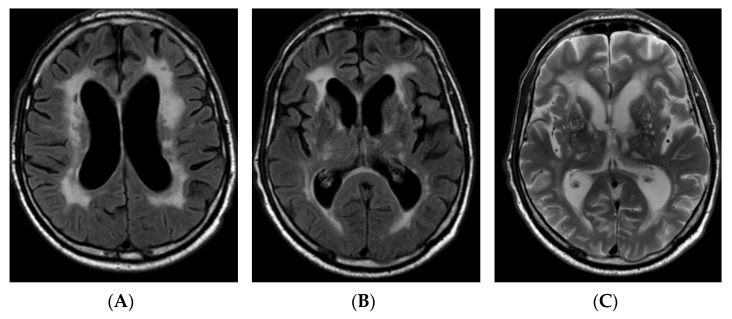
FLAIR axial images (A,B) and axial T2-weighted image (C) of brain MRI, performed after 3 years of initial brain MRI, demonstrating more subcortical and periventricular T2/T2 dark-fluid hyperintensities, Fazekas scale score 3, new lacunar lesions in right thalamus, progressive ventricular dilation (HI—82). FLAIR: fluid attenuated inversion recovery; MRI: magnetic resonance imaging; HI: Huckman index.
